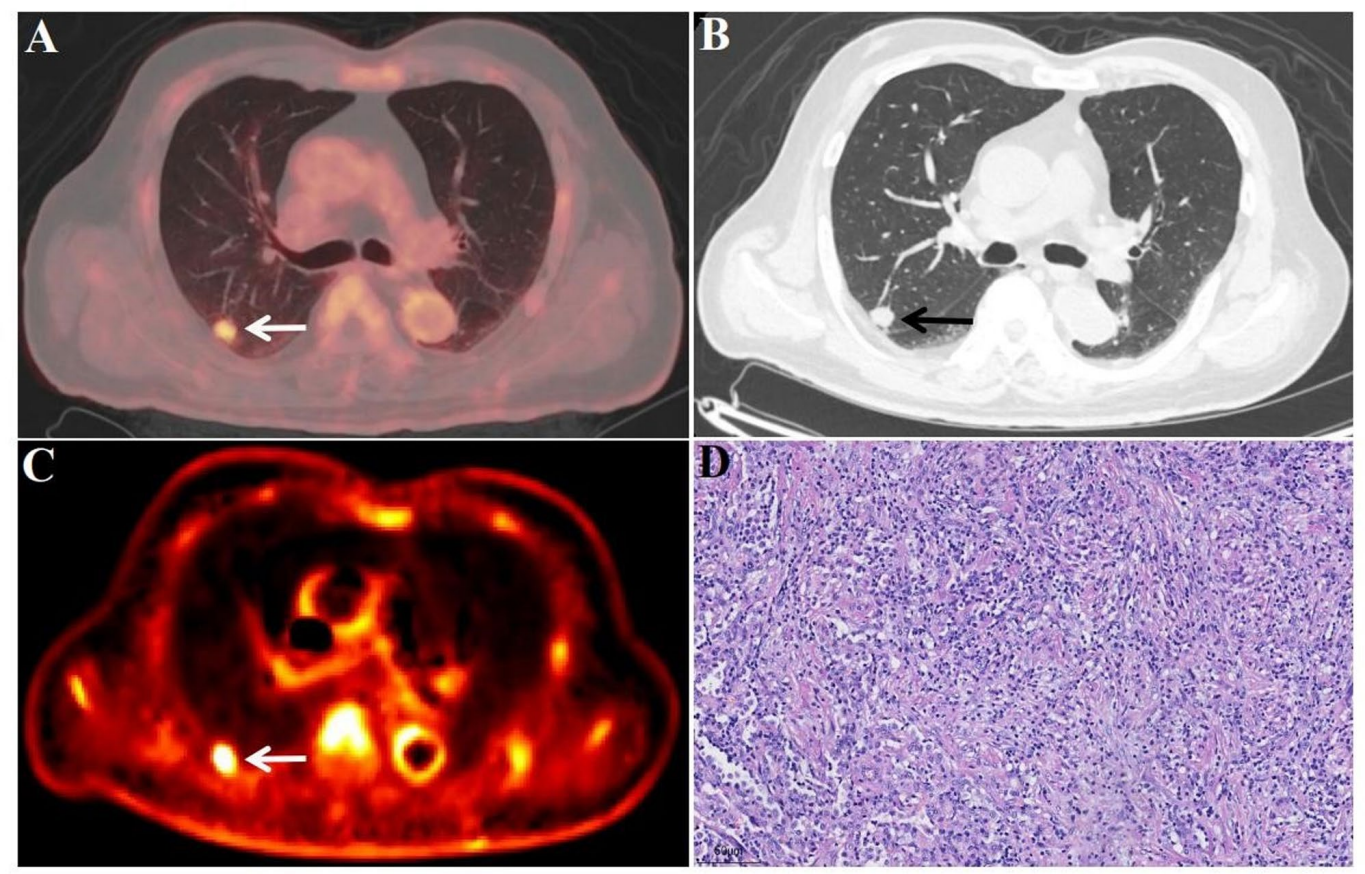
FDG-PET/CT images of a benign lesion A 66-year-old male patient. Surgical pathology confirmed an inflammatory lesion (D, 20x field of view) in the lower lobe of the right lung (white/black arrow), with a size of 1.4 × 1.2 cm (A and B, white/black arrow), SUV_max_ of 4.1 (A, white arrow), and K_i_ of 0.0102 ml/g/min (C, white arrow)
